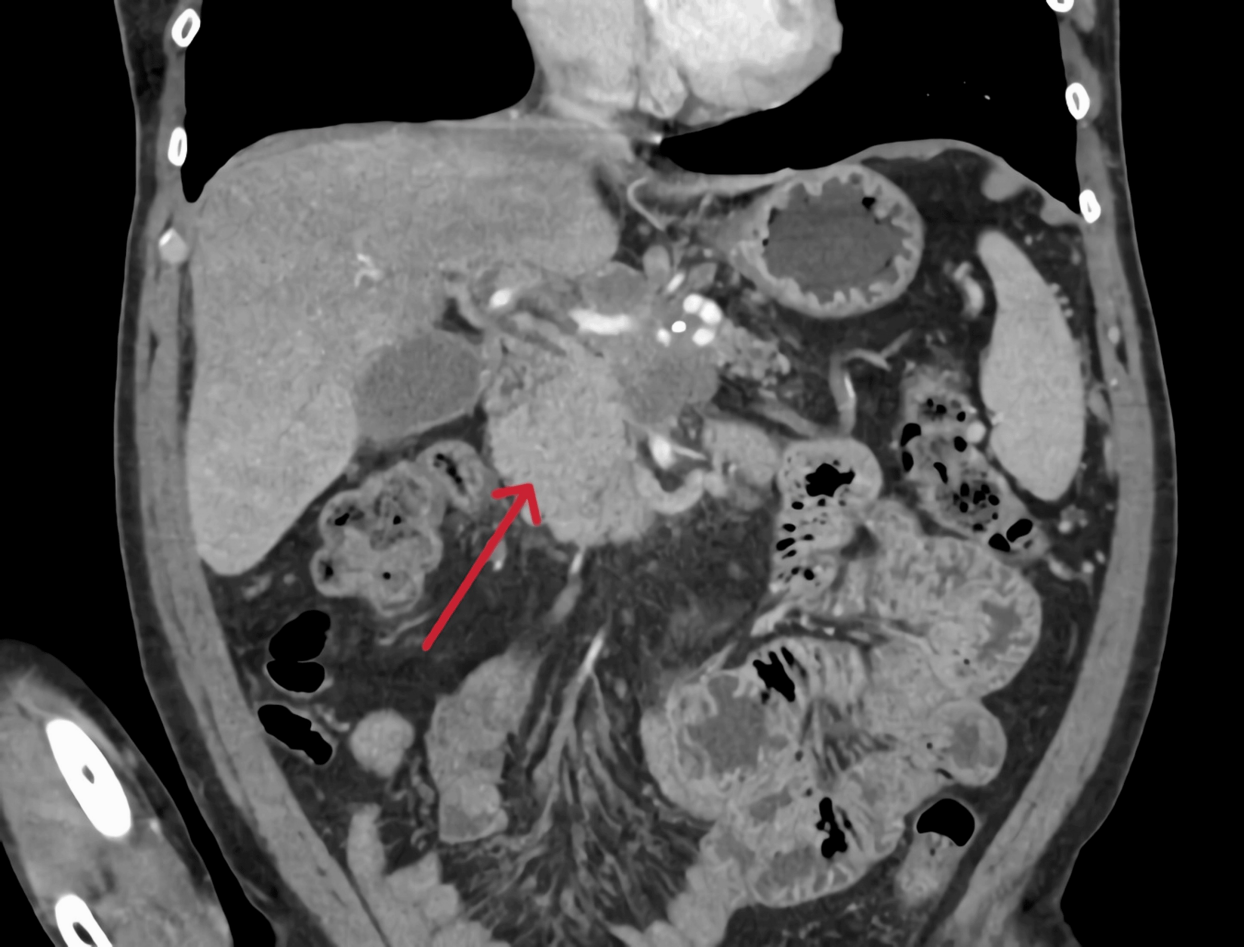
Abdominal-pelvic CT scan Hypodense mass centered in the body of the pancreas, highly suggestive of a locally advanced primary malignant pancreatic tumor
